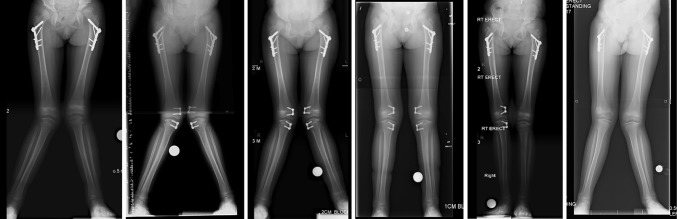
X-rays showing ‘typical’ improvement in knee alignment with insertion of eight-plates and recurrence of deformity following plate removal
